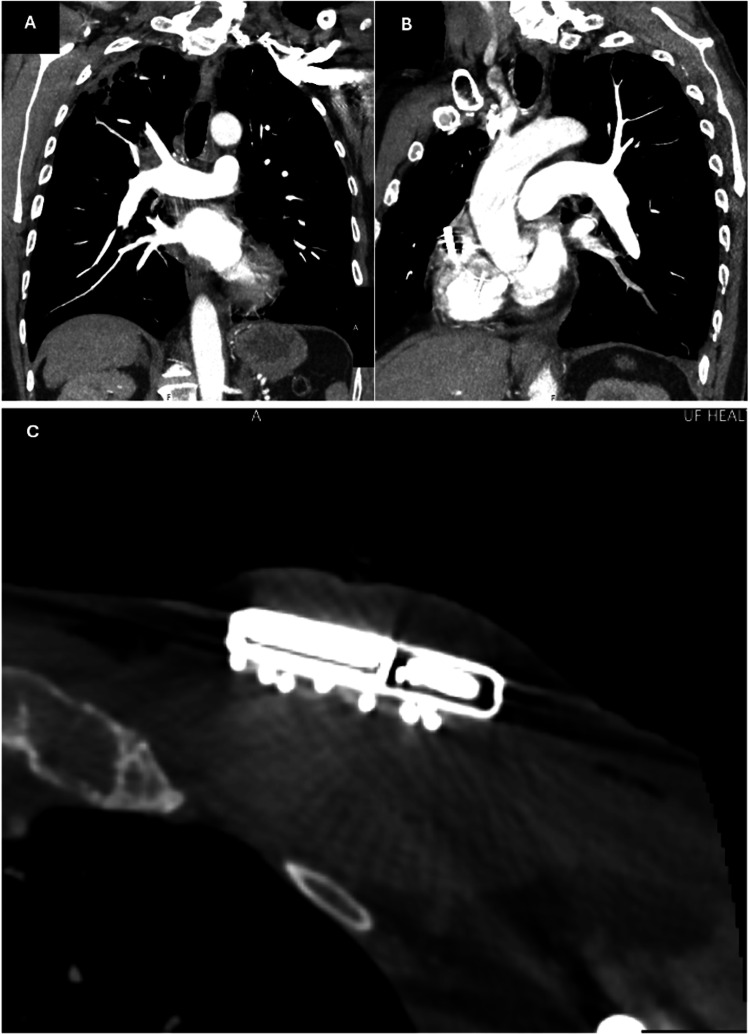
Computed Tomography Angiogram (A,B) CT angiogram, coronal view, demonstrating a lack of acute thrombus within the right and left pulmonary system, respectively. (C) CT angiogram, transverse view, demonstrating a lack of soft tissue edema or fat stranding.
